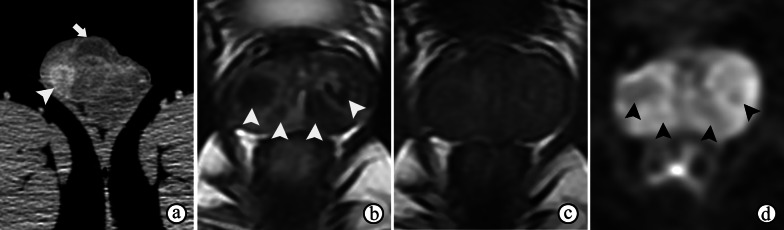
Genitourinary tuberculosis in a 26-year-old man with a right scrotal skin ulceration accompanied by pus discharge and a strongly positive tuberculin skin test. a Computed tomography image shows an enhanced nodule in the right epididymis (arrowhead) with testicular hydrocele (arrow). b T2WI shows prostate atrophy and multiple extremely hypointense nodules inside the tissue (arrowheads). c T2WI shows slightly inhomogeneous signal intensity of the prostate. d DWI at a b-value of 800 s/mm^2^ shows slight hypointensity of the nodules (arrowheads)
